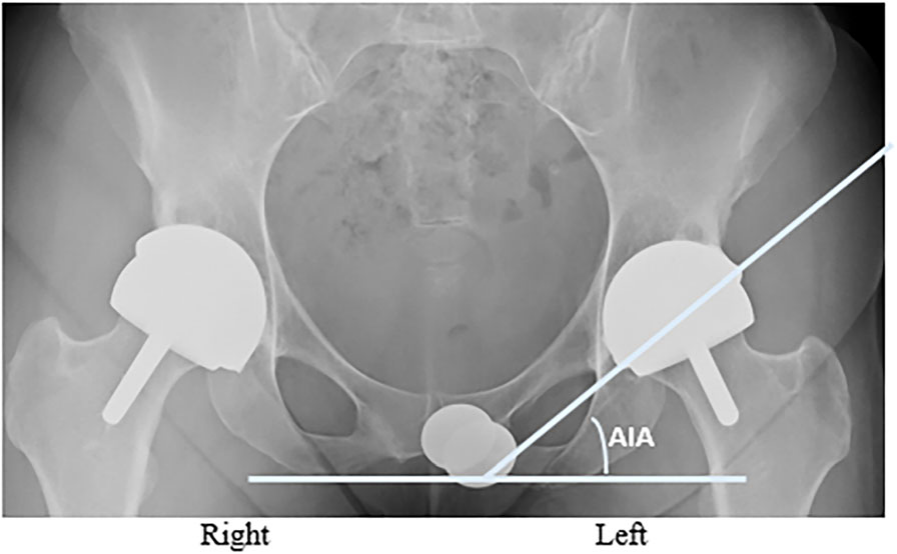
Pelvic radiograph acetabular inclination angle measurement lines. Anterior-posterior pelvis radiograph taken 5 years after a hybrid metal-on-metal Corin hip resurfacing on the right hip and 2 years after a hybrid metal-on-metal Biomet ReCap™ hip resurfacing on the left hip. Better AIA is noted in the most recent HRA on the patient’s left side
